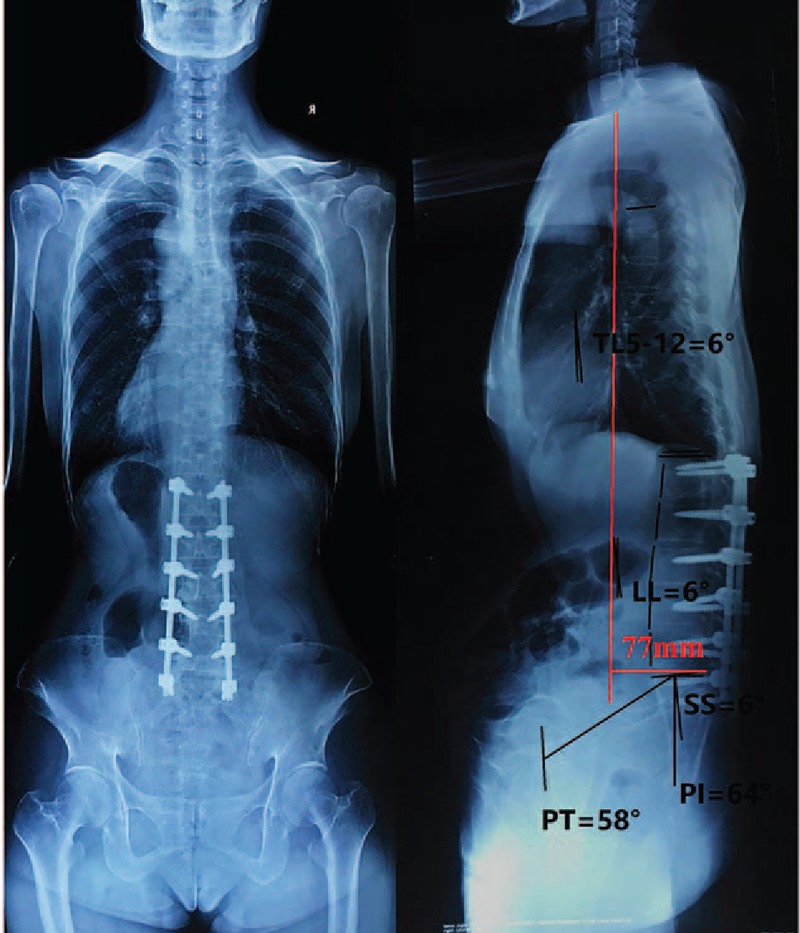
Positive and lateral full spine x-ray 2 weeks after surgery. PI = 64°, PT = 58°, SS = 6°, LL = 6°, T5-T12 = −6°, SVA = 77 mm. PI = pelvic incidence, PT = pelvic tilt, SS = sacral slope, SVA = sagittal vertical axis from C7 plumb line.
